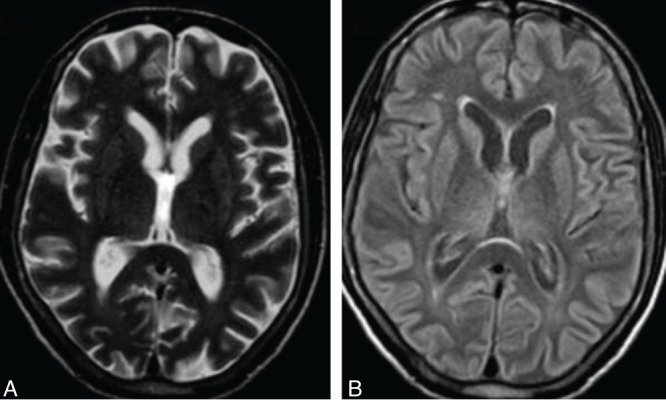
Axial T2 (A) and FLAIR (B) images show no abnormal T2/FLAIR hyperintensities in the basal ganglia, thalami, and both cerebral hemispheres, indicating no extrapontine abnormality on the present scan.
